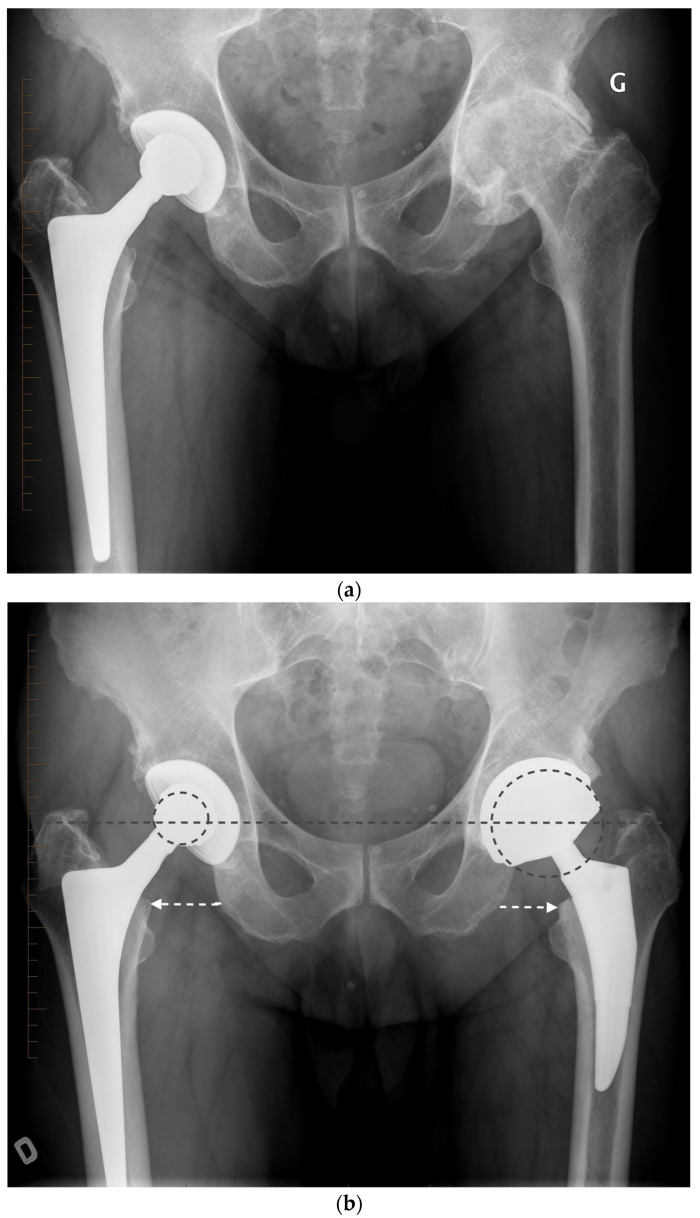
(a) Antero-posterior pelvis radiograph of a 55-year-old man with a right metal-on-metal 28 mm THA and severe osteoarthritis of the left hip. (b) Postoperative radiograph after a left LDH THA with leg length and femoral offset restoration.
